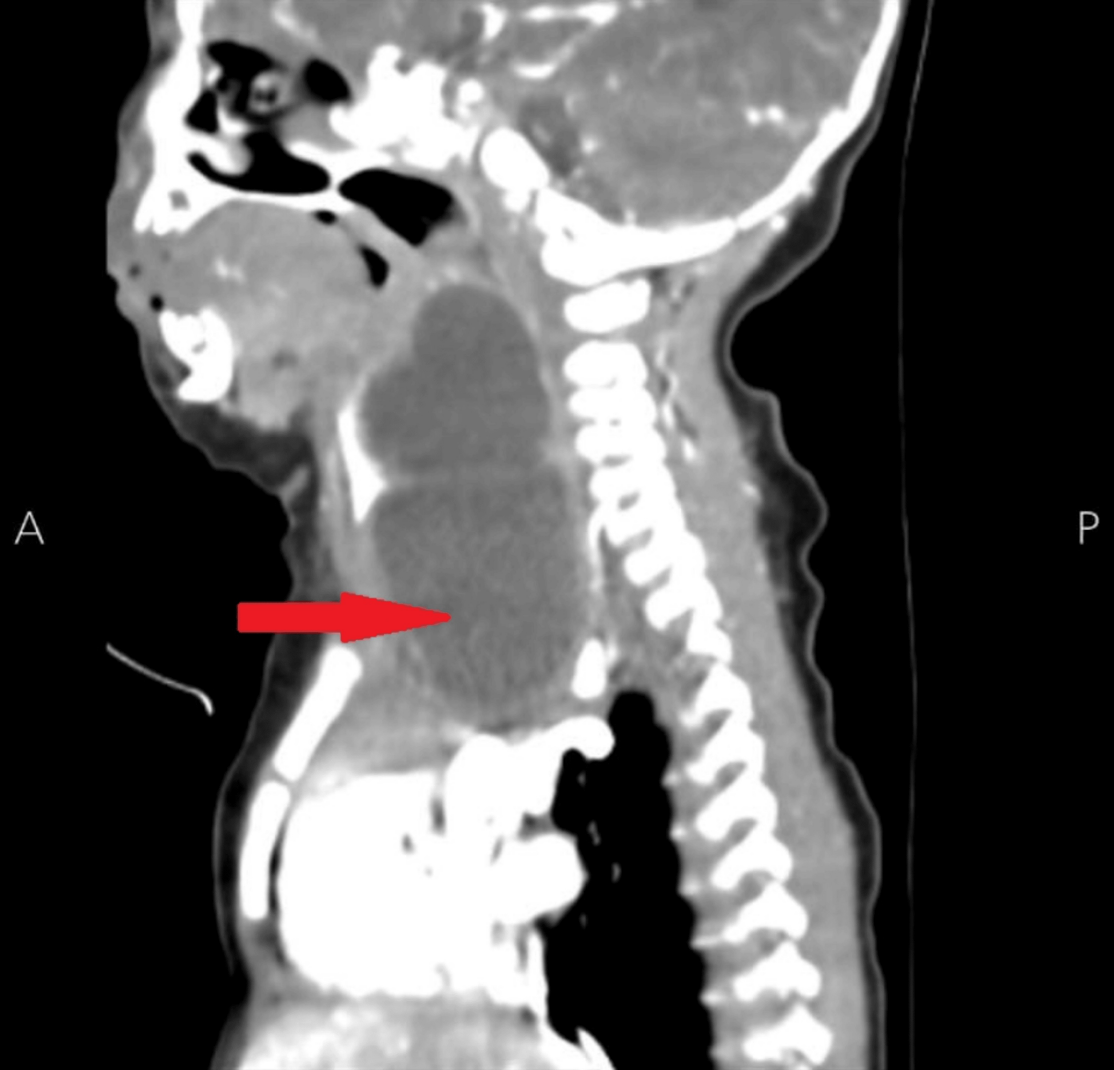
The sagittal section of the contrast-enhanced CT scan shows 3.5×3.1×5.6 cm of cervical mass extending to the mediastinum, shifting cervical structures, and causing a mass effect (red arrow). CT: computed tomography
